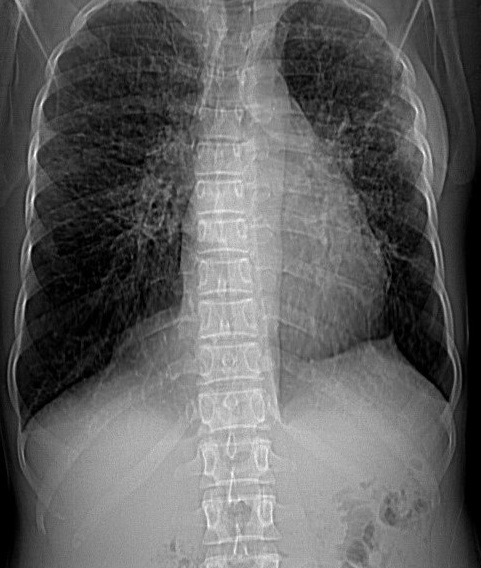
Chest CT scan scannogram reveals severe honeycombing especially in the middle and upper lobes of both lung fields from combination of tramline and bronchial thickening
